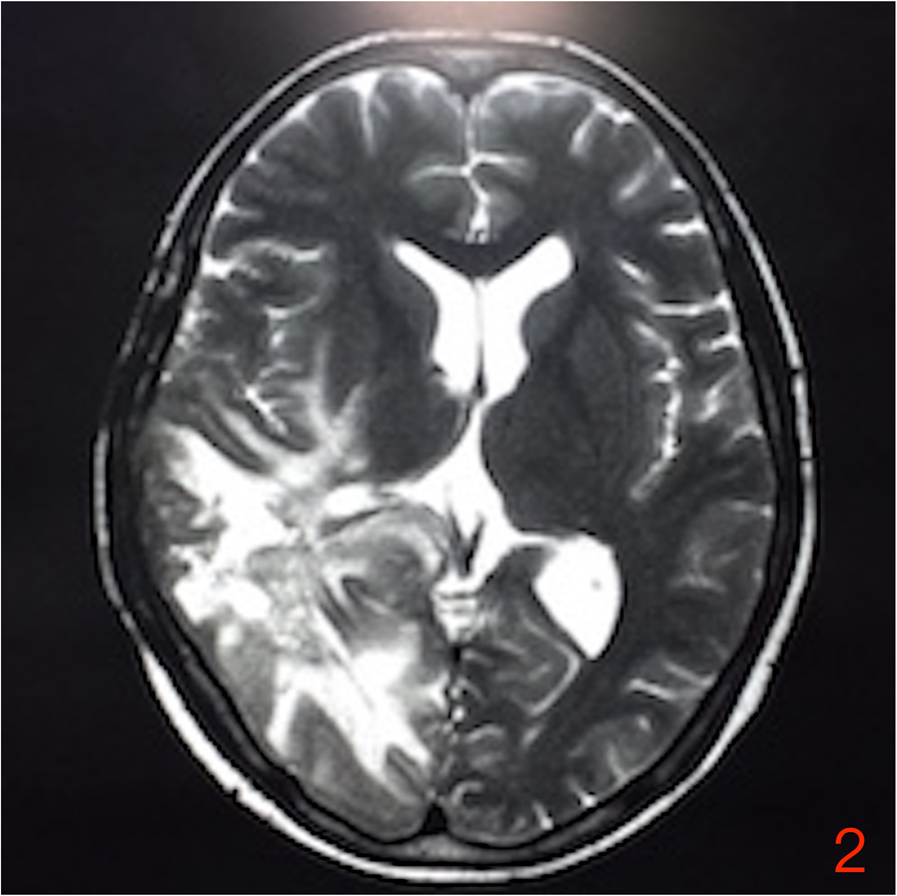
Head MRI showing multiple occupations in the right temporal, apical, and occipital lobes and in the corpus callosum
